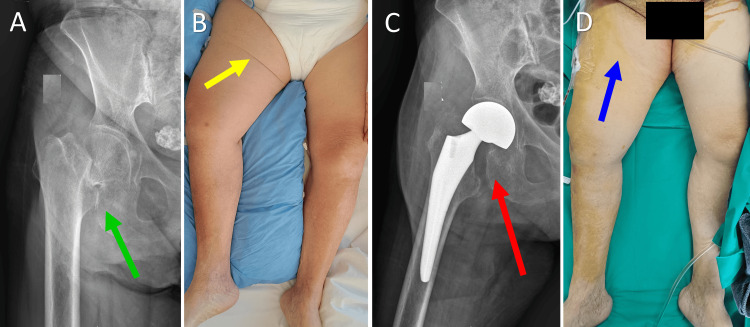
Asymmetric skin fold (ASF) formation in a 76-year-old woman after a femoral neck fracture A. An anteroposterior pelvic X-ray indicating the femoral neck fracture of the right hip (green arrow); B. The ASF formation on the right thigh is due to proximal migration of the right femur and subsequent limb shortening (yellow arrow). C. An anteroposterior X-ray indicating a hemiarthroplasty of the right hip (red arrow); C. The ASF disappeared after fracture treatment and restoration of limb length (blue arrow). Image credits: P. Samelis
